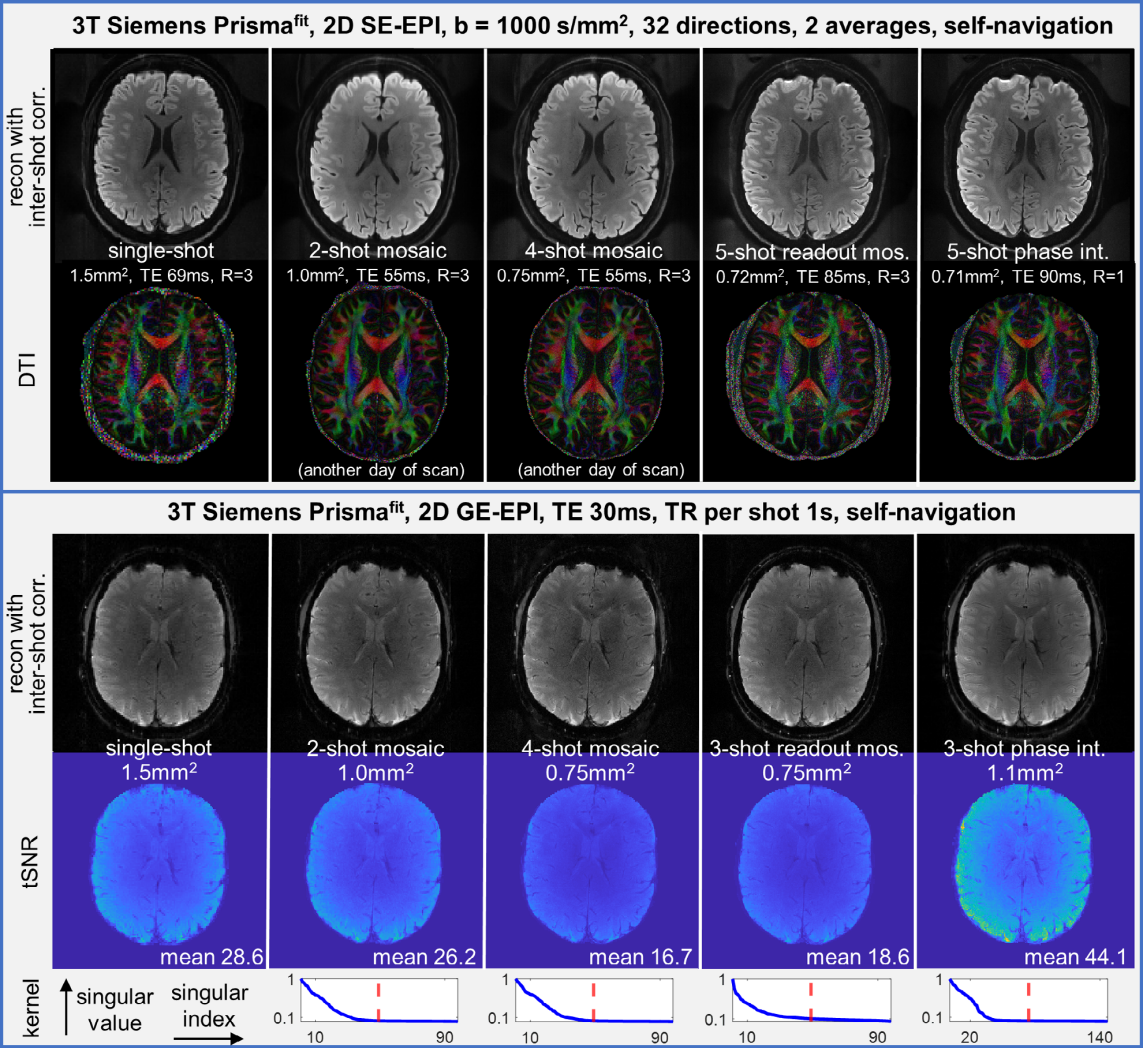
DWI, DTI, GE‐EPI images with the four self‐navigated EPI sequences. Upper: The DWI/DTI were acquired with 2D SE‐EPI with 1000 s/mm^2^, 32 diffusion directions, and 2 averages. Their ESPs are the same as the sequences in Figure 5. The 2D navigator was removed and the time duration of one‐shot‐one‐slice acquisition became shortened by at least a few tens of milliseconds, as documented in Supporting Information. The two‐shot and four‐shot mosaic diffusion scans were taken on another day of separate scan with smaller slice number and shim volume, due to sensitivity to *B*
